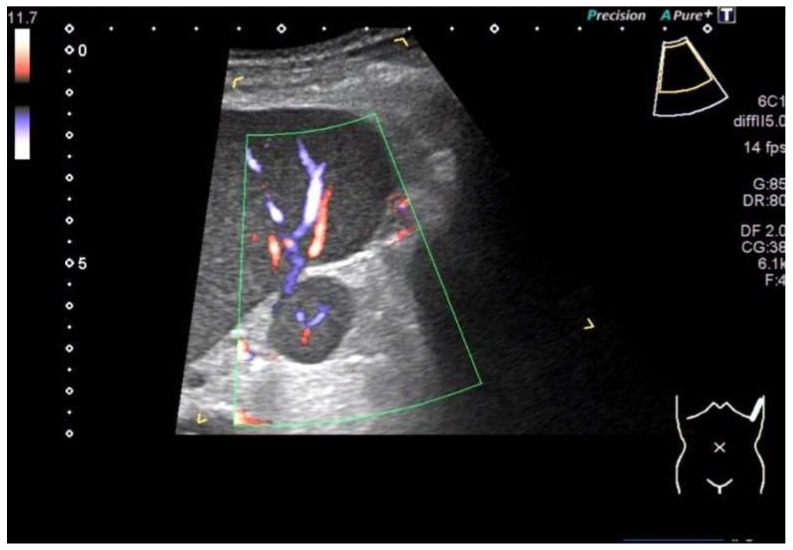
A typical case of an accessory spleen located between the hilum and the lower pole of the spleen. B-Mode ultrasound shows a roundish solid focal lesion of 2 × 1.8 cm with a smooth outline, which has the same echogenicity and echopattern as the spleen. Color Doppler ultrasound shows a centrally branching accessory splenic artery and intrasplenic arterial and venous vessels.
